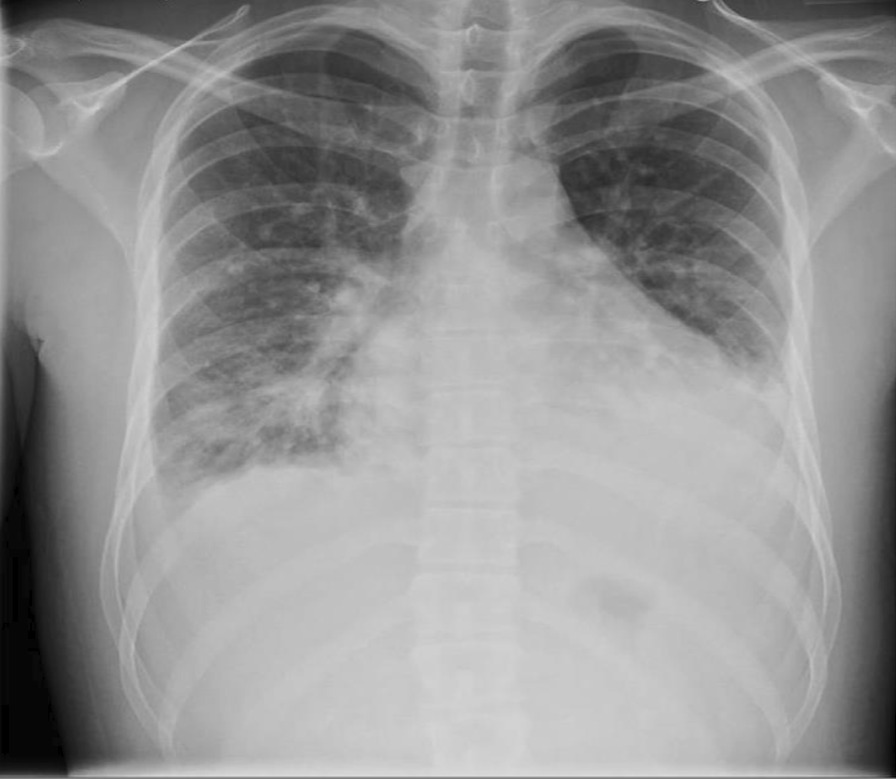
A portable chest x-ray demonstrating bilateral interstitial infiltrates with dense consolidations in lung bases, bilateral pleural effusions, and pericardial effusion
